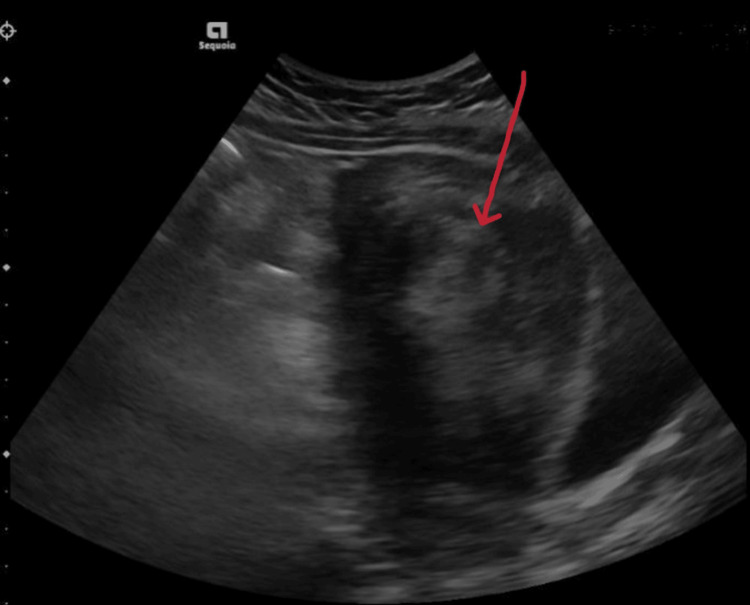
Transvaginal ultrasound showing solid appearing lesion in right adnexa (red arrow)
